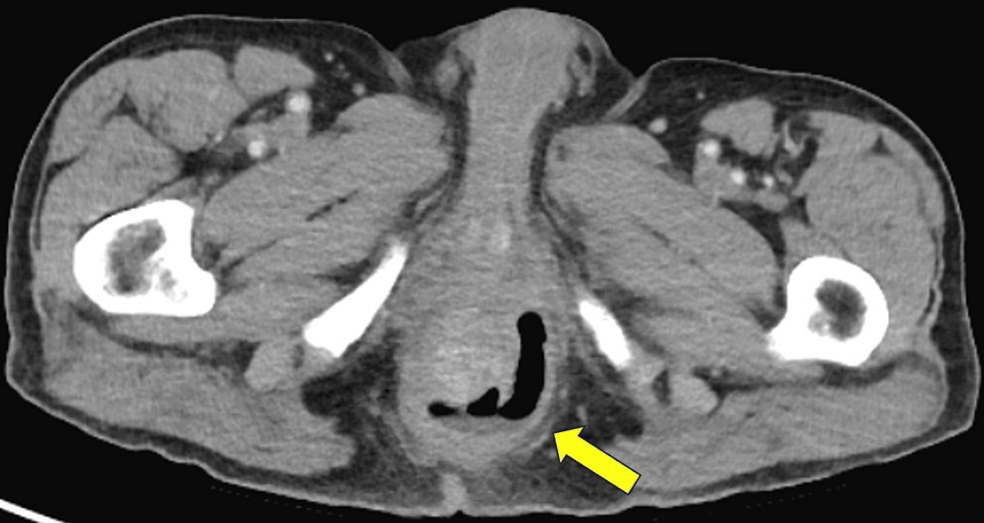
CT of the abdomen/pelvis with IV contrast A large complex horseshoe perirectal abscess containing fluid and air with marked thickening of the rectum is seen.
